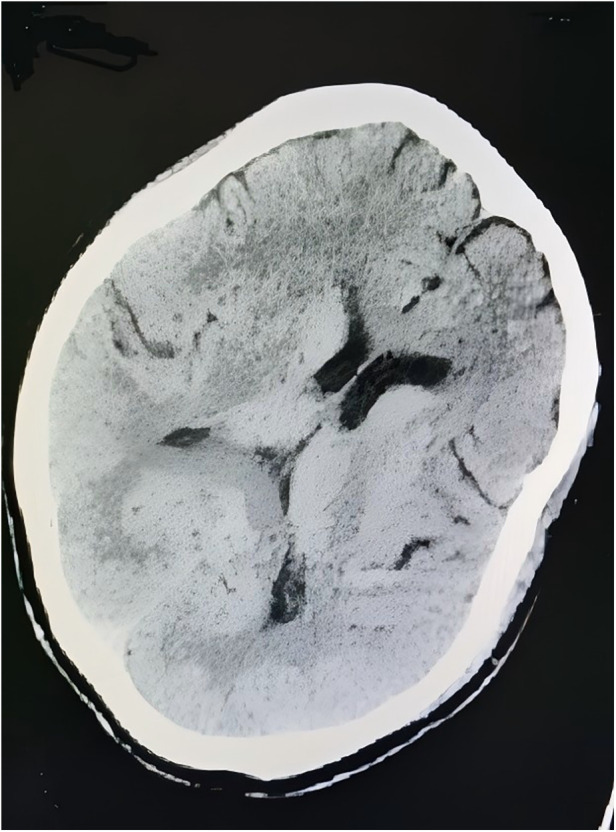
Urgent head computed tomography scan demonstrating no intracranial abnormalities 30 min after stroke onset.
